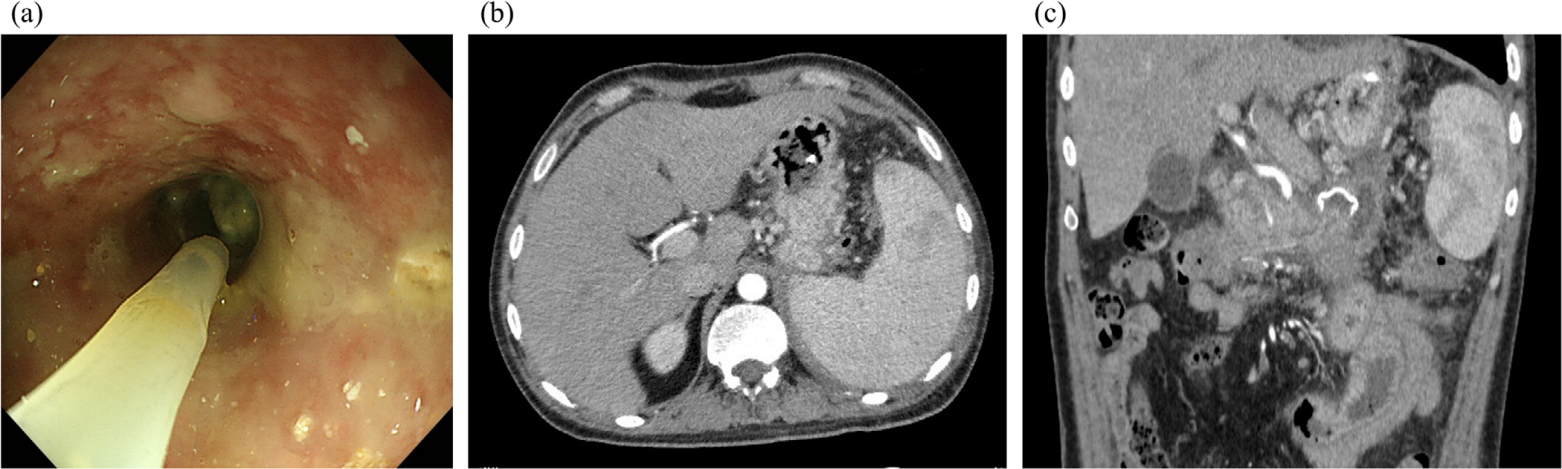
Endoscopic view and abdominal contrast‐enhanced computed tomography (post‐treatment). (a) The condition is greatly improved by endoscopic necrosectomy and drainage. (b, c) The walled‐off necrosis has almost completely disappeared
